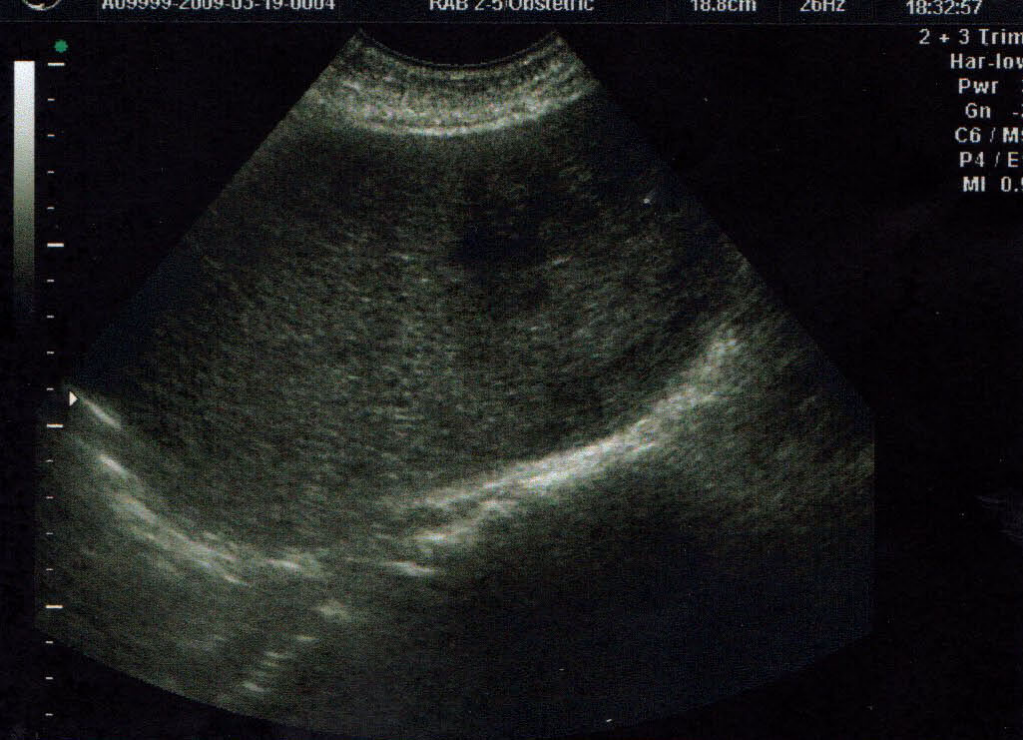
Ultrasound showing uterus content compatible with molar pregnancy.
